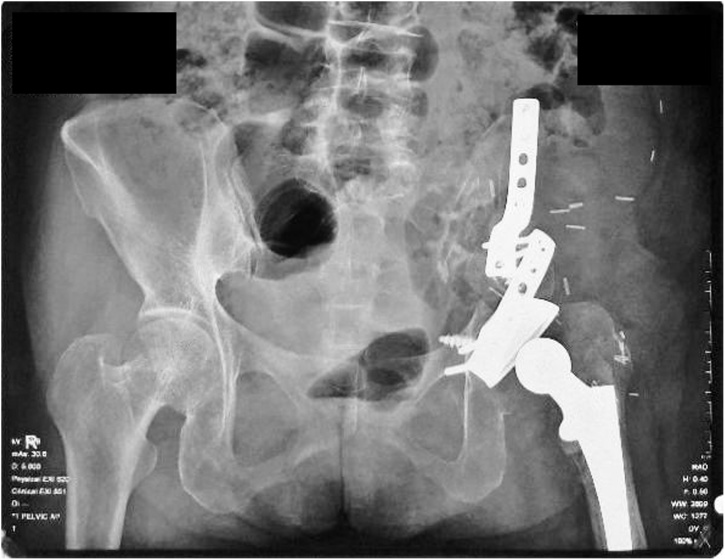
Postoperative Pelvic X-Ray Examination.
